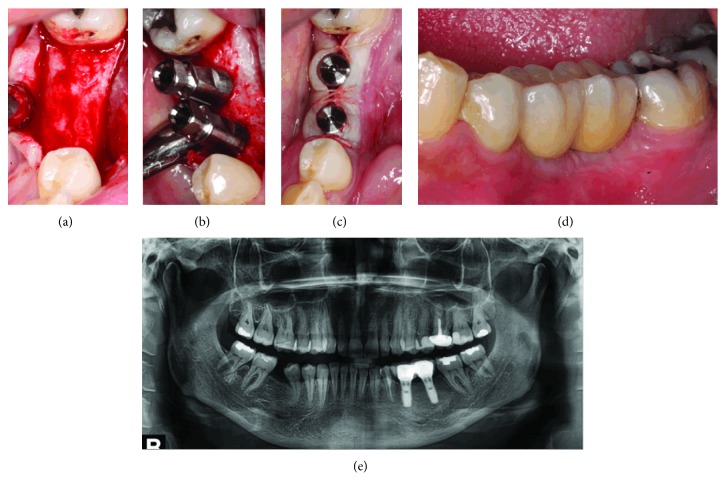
(a) Clinical view of the bone healing before implant placement. (b) Two Swiss Plus Zimmer® implants placed in postalveolar locations of teeth 35 and 36. (c) Clinical aspects of cover screws and sutures, immediately after implants placement. (d) Clinical aspect of restored implants (35 and 36). (e) Panoramic radiograph showing the final situation of the bone and the restored implants.
